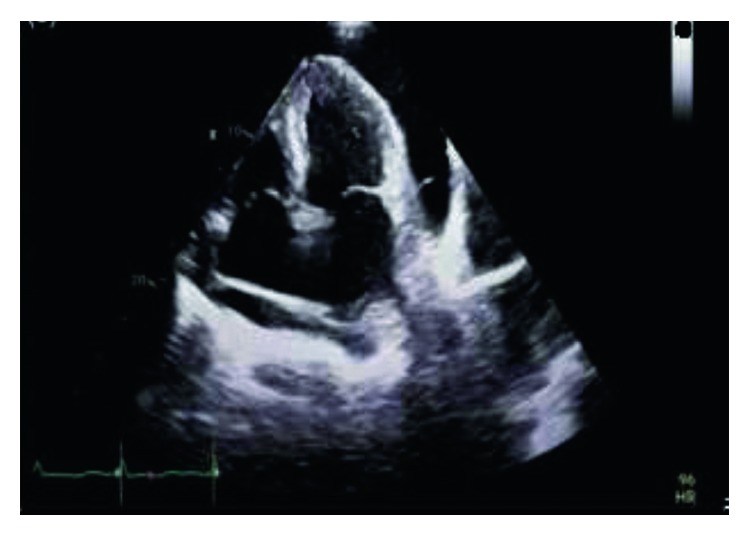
Two-dimensional TTE shows pericardial effusion in apical 4-chamber view.
